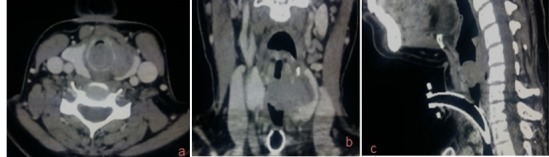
Contrast-enhaced cervical computed tomography: axial (A), coronal (B) and sagittal view (C): tumoral lesion between the left thyroid lobe, the left edge of the subglottic area of the larynx and the first tracheal ring filling all the lumen. The origin could be either the thyroÃ¯d gland or the larynx
